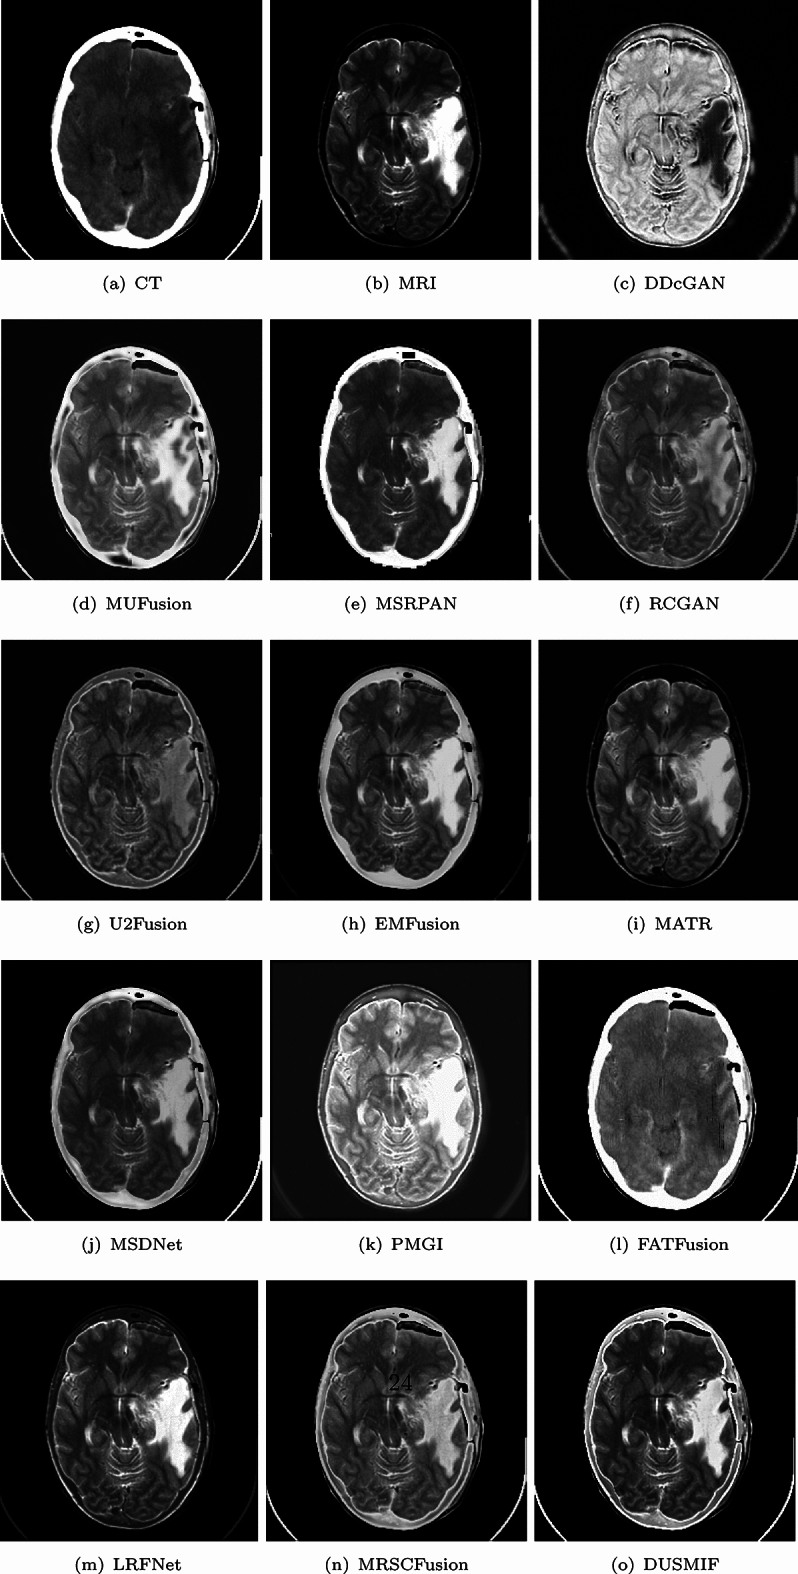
The second comparative display of CT-MRI medical image fusion.
